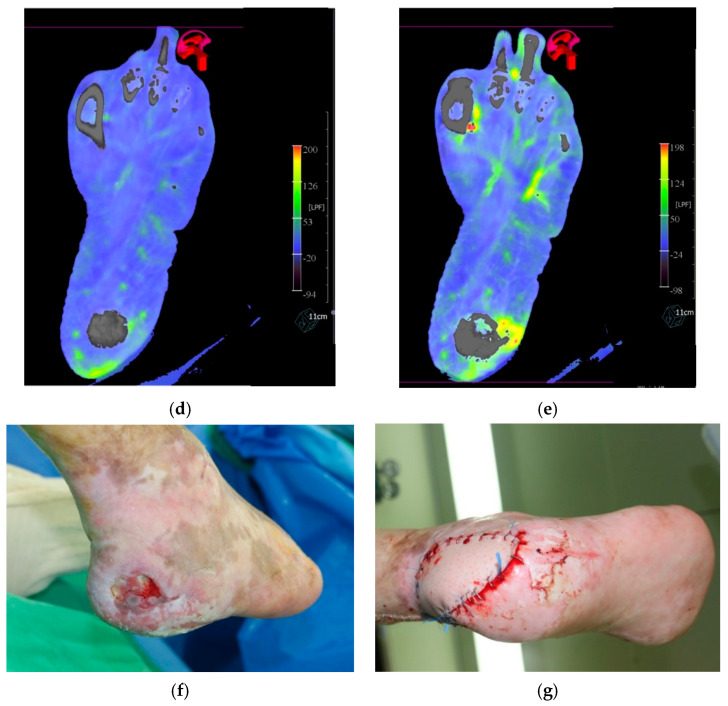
A 58-year-old man with peripheral arterial disease underwent percutaneous transluminal angioplasty (PTA) for heel wound. (a) Initial angiography shows total occlusion of left dorsalis pedis artery. (b) Recanalization with 0.014-inch guidewire and balloon angioplasty was done with a 1.5 mm–4 cm balloon catheter. (c) Completion angiography shows patent left dorsalis pedis artery and no significant change of flow to heel side. (d,e) Cone-beam computed tomography-based foot perfusion mapping before (d) and after (e) PTA clearly shows markedly increased perfusion of the foot, especially heel side. (f) Photography shows a wound on the heel side. (g) Patient underwent reconstruction surgery with an anterolateral thigh flap two weeks later after PTA without undergoing amputation.
